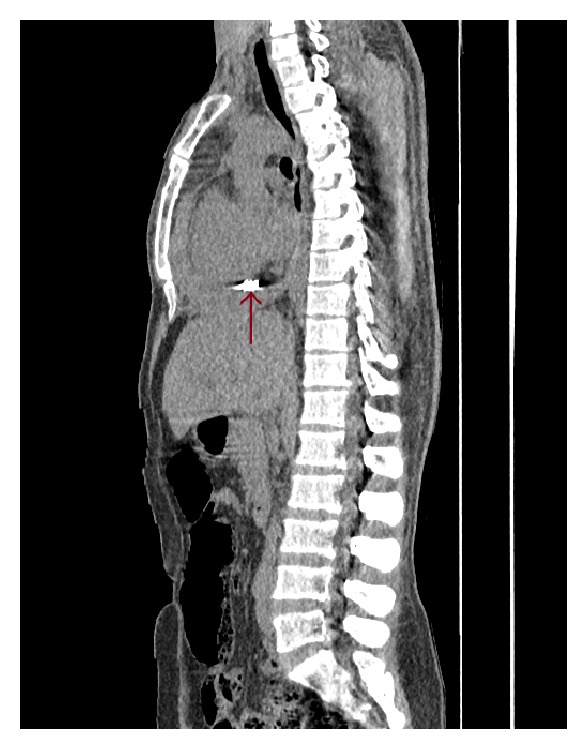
Sagittal cut view of chest CT shows a bullet in the pericardial sac with pericardial effusion.
